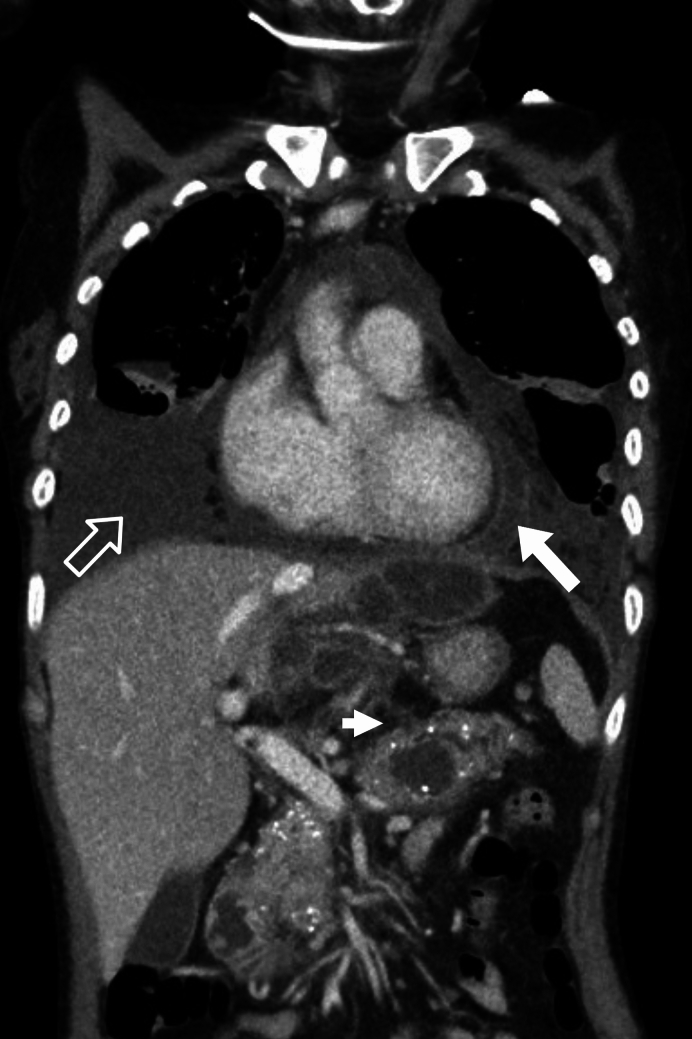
CT demonstrated a fistula tract extending from the body of the pancreas into the right pleural space. Amylase rich fluid in pericardium (bold arrow). Amylase rich fluid in right pleural cavity (open arrow). Location of the fistula (short arrow)
